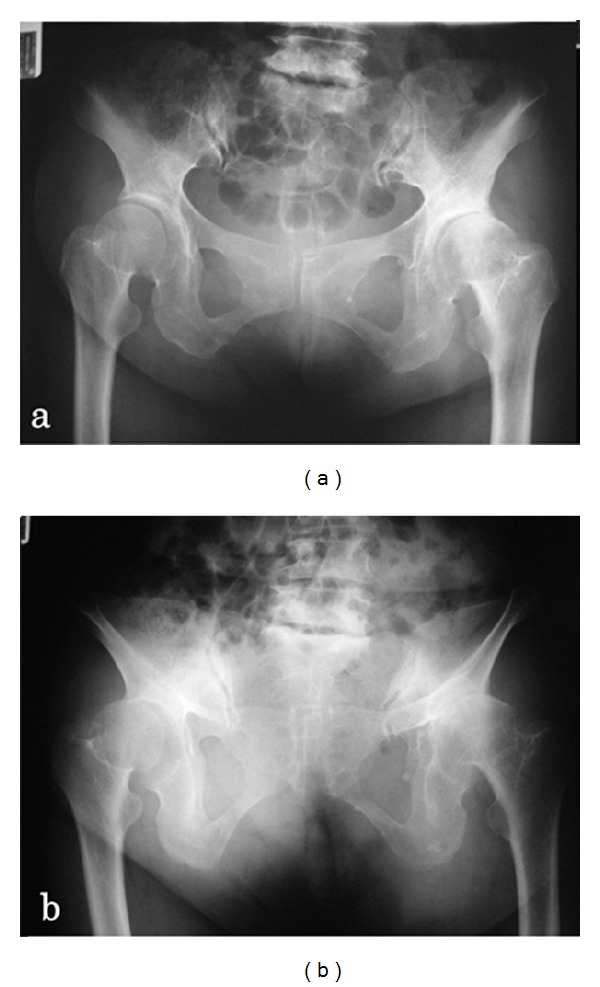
Anteroposterior radiographs of the bilateral hip joints on lying (a) and standing (b). Plain radiography showed osteoarthritic changes of hip joints and severe posterior pelvic tilt on visualizing superior displacement of both femoral heads.
